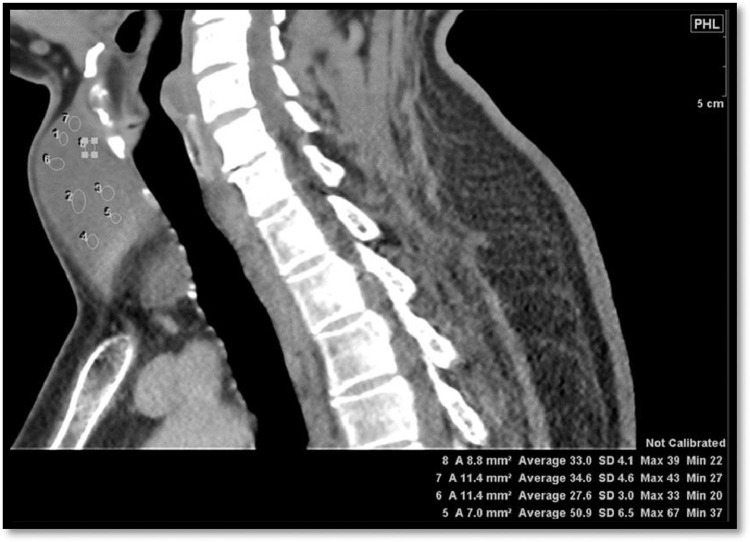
Sagittal CECT neck showing CT attenuation taken at different points of the colloid cyst (varied between 27-51 Hounsfield Unit.
